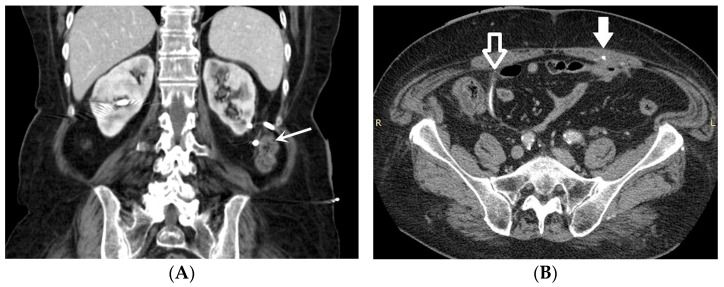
Bilateral nephrostomy. Coronal CT image (A) and axial MIP image (B). The left-sided catheter is dislodged outside of the urinary tract (arrow).
